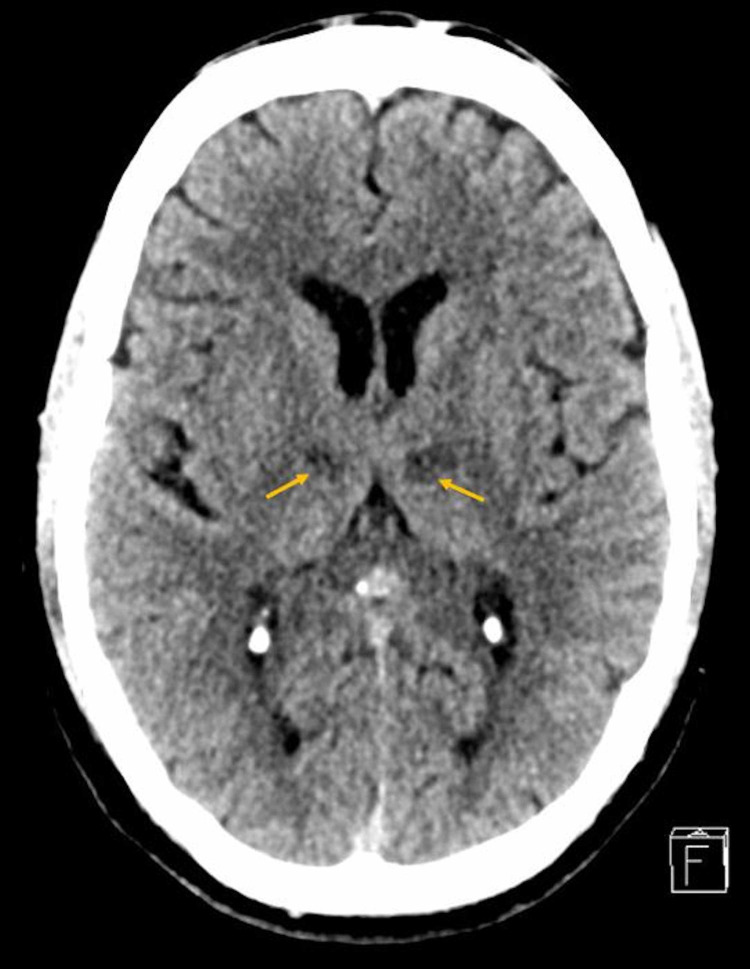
Brain plain CT axial image.
